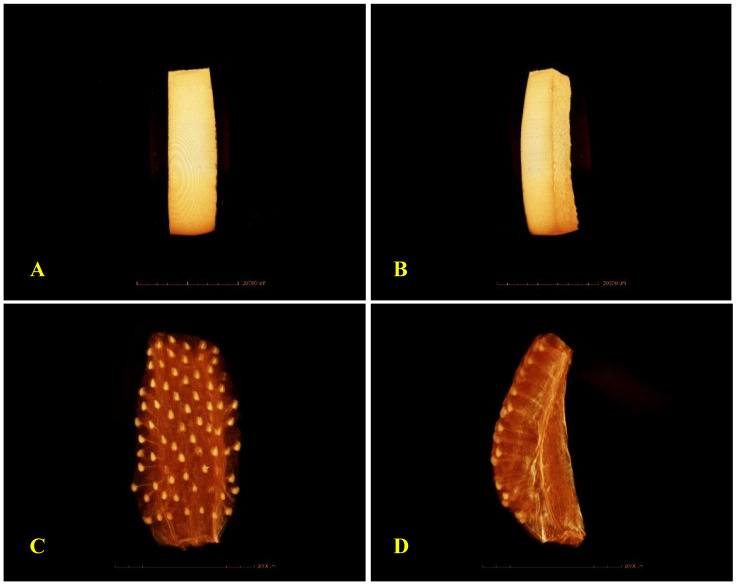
µ-CT scan microimages of external (A) and internal (B) tissue structure of fresh bell pepper, and external (C) and internal (D) tissue structure of fresh strawberries.
